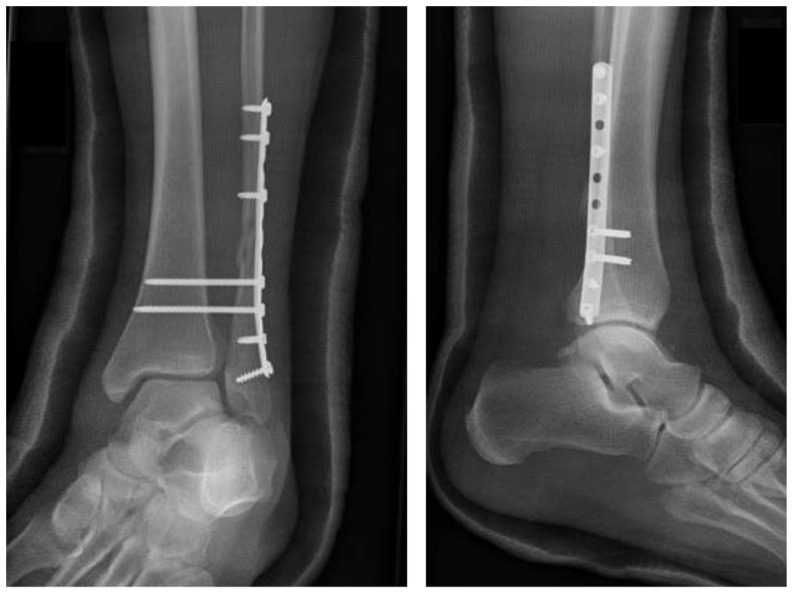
Postoperative radiographs at 6 weeks showing lateral-only fixation using 1/3rd tubular plate and syndesmosis screws with maintenance of alignment and early healing of distal fibular fracture.
